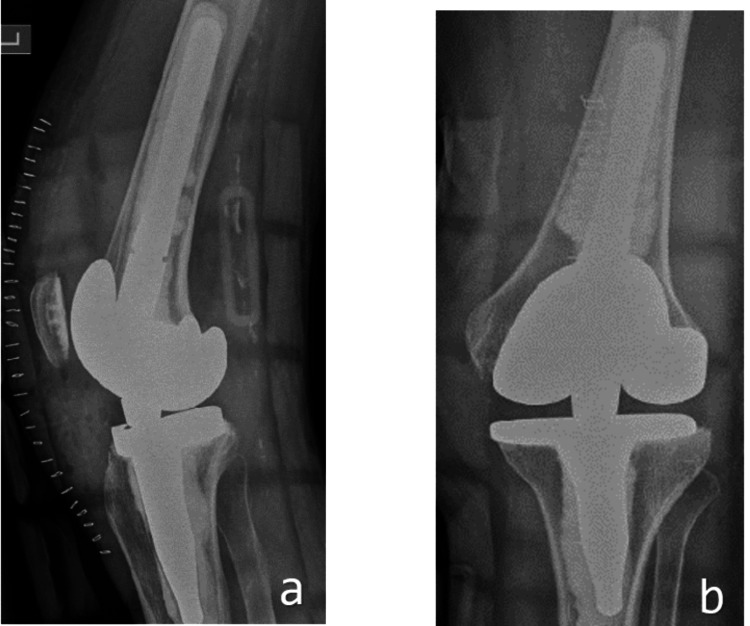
Postoperative anteroposterior (a) and lateral (b) X-rays showing rotating hinge (NexGen, Zimmer) total knee replacement with deformity correction
